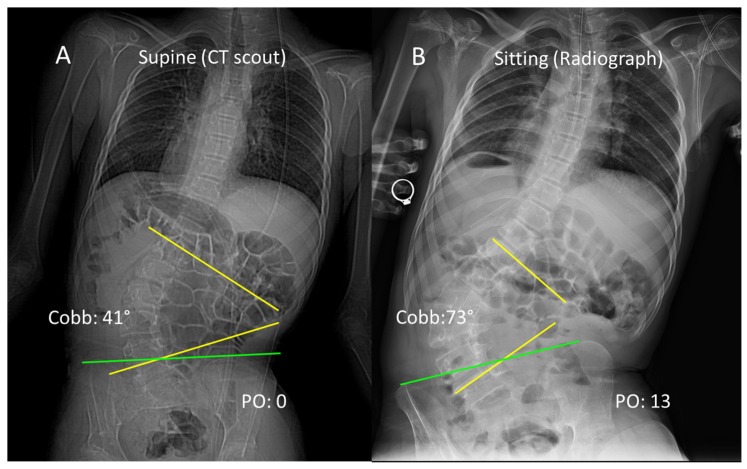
Positional Dependency of Radiographic Measurements in Non-ambulatory Patients. > Comparison of supine (A) and unsupported sitting (B) coronal imaging in a GMFCS V patient, obtained on the same day. Image (A) is a supine computed tomography (CT) scout view, used to minimize additional radiation exposure, and shows an artificial correction of the curvature and pelvis due to the removal of gravity. Image (B) is a sitting posteroanterior (PA) radiograph revealing the true functional trunk collapse and significant pelvic obliquity. This emphasizes the risk of relying solely on supine imaging, which can hide the criteria for surgical referral. Abbreviations: PO, pelvic obliquity. The yellow lines represent the measurement of the Cobb angle, and the green line indicates pelvic obliquity.
